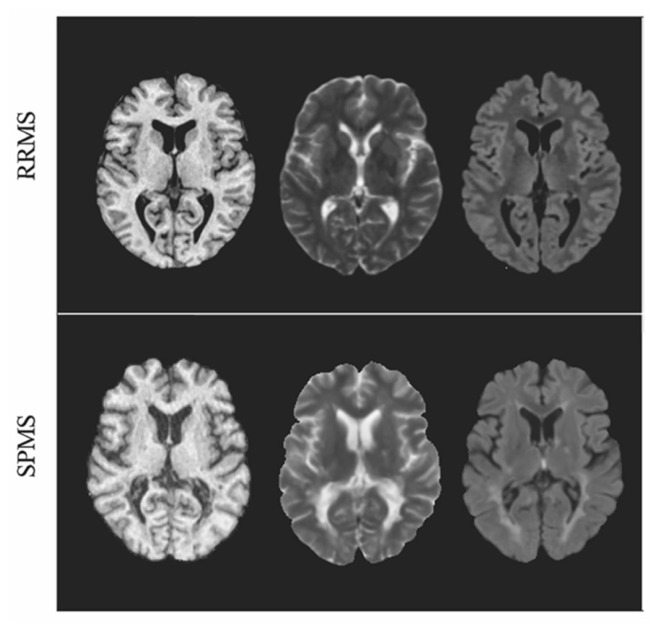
Example brain MRI slices from two correctly classified subjects. Shown from left to right are T1-weighted, T2-weighted, and FLAIR MRI. The RRMS subject was a female, with an onset age at 40s (50s years old at scan), EDSS score of 1.0, and disease duration of 13 years at scan. The SPMS subject was a male, with an onset age at 30s (40s years at scan), EDSS score of 3.0, and disease duration of 14 years.
